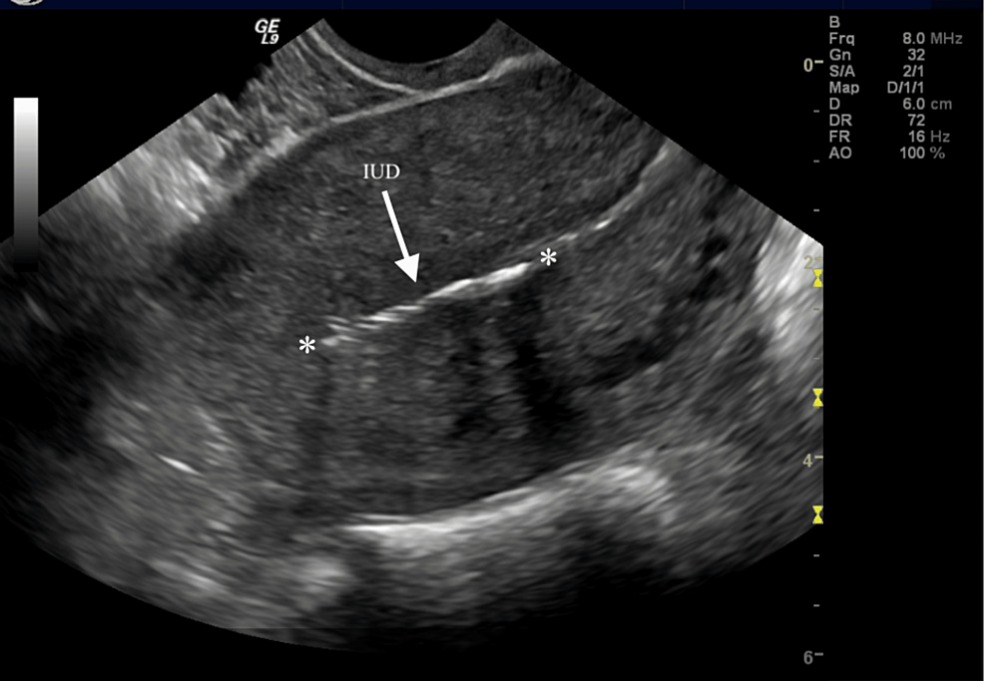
Ultrasound of the IUD in proper position within the uterus at the time of initial placement. IUD = intrauterine device
